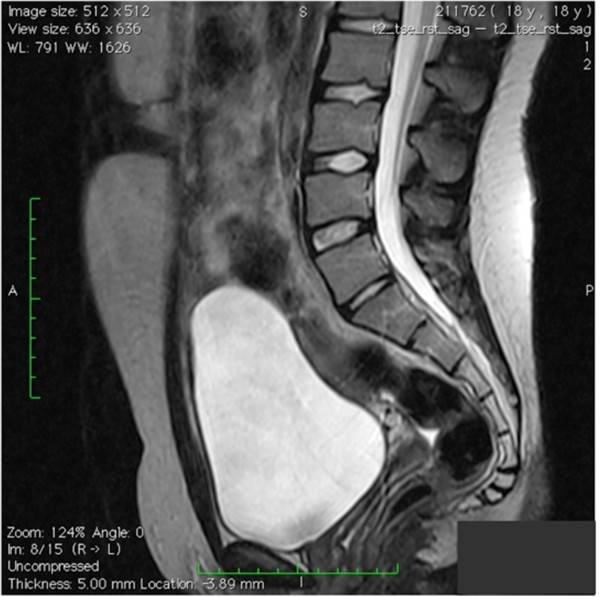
Magnetic resonance imaging scan showing the absence of the urachal cyst 6 years after surgery.
